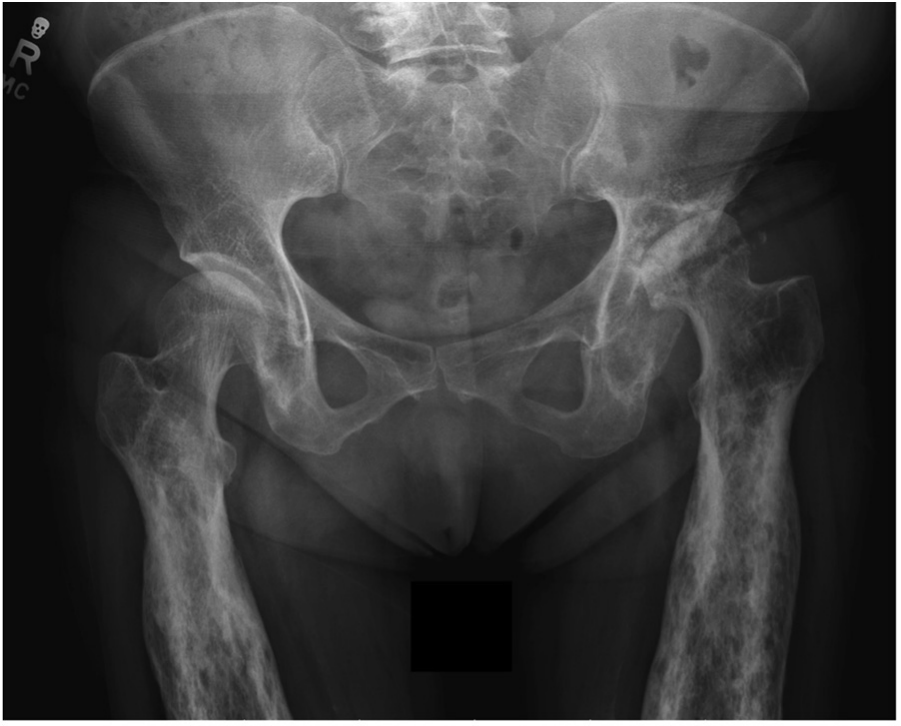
Preoperative anteroposterior pelvis radiograph showing advanced degenerative changes to the left hip with a large subchondral cyst in the roof of the acetabulum and a Paprosky IIB defect in the lateral rim of the acetabulum. Proximal and lateral migration of the left hip is also noted, consistent with Crowe II dysplasia.
